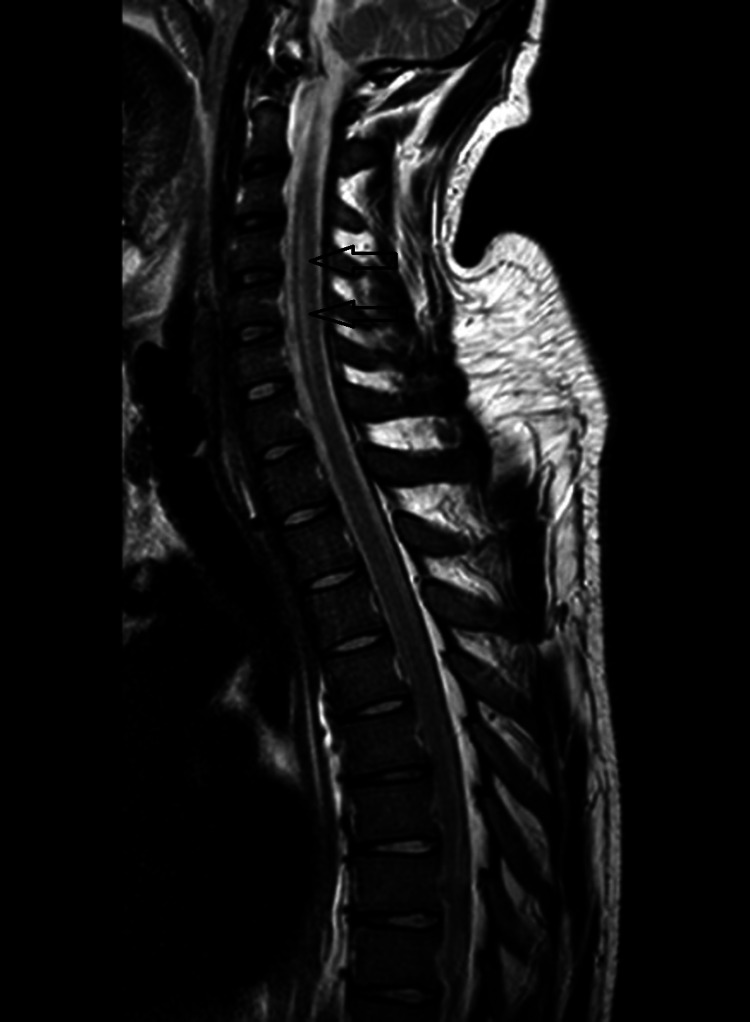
CT scan of sagittal view of cervical and thoracic spine showed T2 signal hyperintensity within anterior horn.
